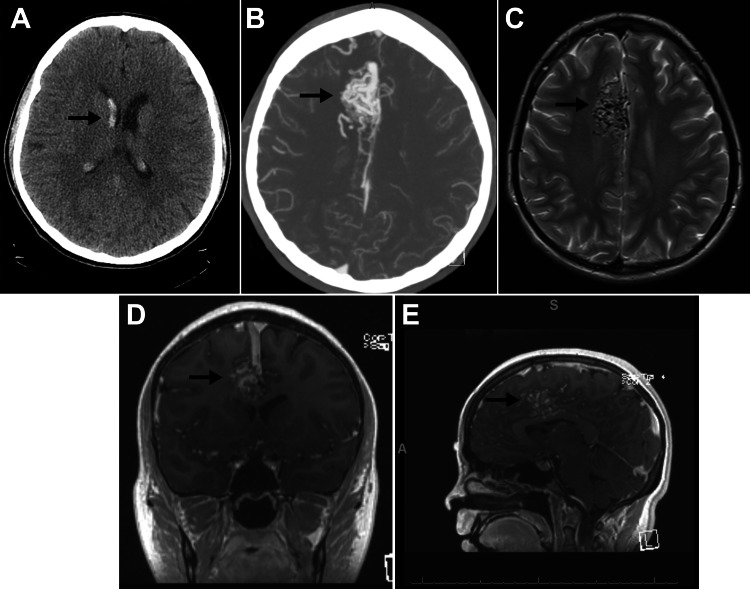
Imaging of intraventricular hemorrhage and AVM. (A) Axial noncontrast CTH demonstrates intraventricular hemorrhage primarily in the right lateral ventricle (arrow). (B) Axial CTA demonstrates a right medial frontal lobe AVM (arrow) with a superficial draining vein. (C) Axial T2-weighted MRI demonstrates the right medial frontal lobe AVM that extends into the SMA (arrow). Coronal (D) and sagittal (E) T1-weighted MRI showing the location of the lesion within the medial right frontal lobe (arrows). AVM: arteriovenous malformation; CTA: computed tomography angiography; CTH: computed tomography of the head; MRI: magnetic resonance imaging; SMA: supplementary motor area
